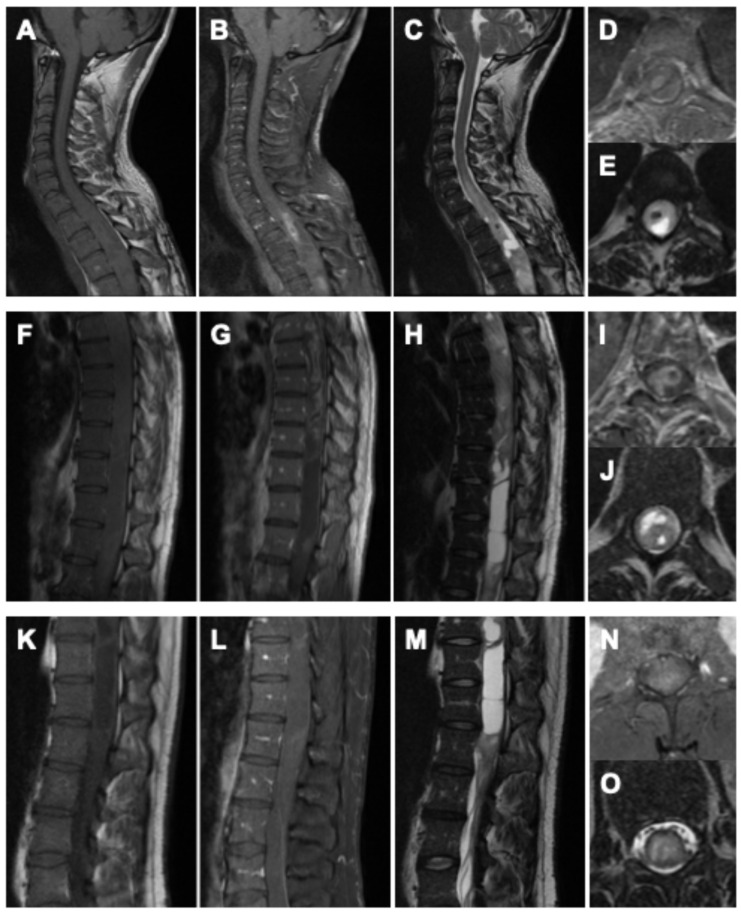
Preoperative imaging of the holocord astrocytoma. Cervical spine: (A) sagittal native T1-weighted MRI, (B) sagittal gadolinium-enhanced T1-weighted MRI, (C) sagittal native T2-weighted MRI, (D) axial gadolinium-enhanced T1-weighted MRI, and (E) axial native T2-weighted MRI. Thoracic spine: (F) sagittal native T1-weighted MRI, (G) sagittal gadolinium-enhanced T1-weighted MRI, (H) sagittal native T2-weighted MRI, (I) axial gadolinium-enhanced T1-weighted MRI, and (J) axial native T2-weighted MRI. Lumbar spine: (K) sagittal native T1-weighted MRI, (L) sagittal gadolinium-enhanced T1-weighted MRI, (M) sagittal native T2-weighted MRI, (N) axial gadolinium-enhanced T1-weighted MRI, and (O) axial native T2-weighted MRI.
